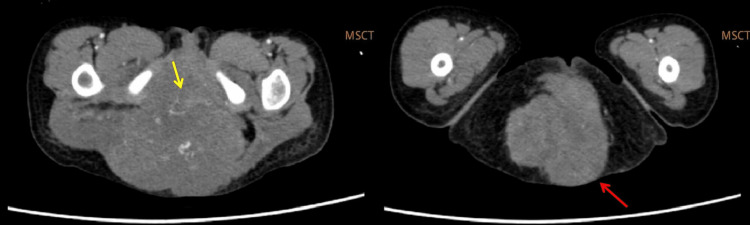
MSCT showed an intraluminal inferior cava vein mass expanding into the pelvic cavity (yellow arrow) and gluteal region with coccygeal osseous destruction (red arrow) with early suspicion of leiomyosarcoma. MSCT, multislice computed tomography
